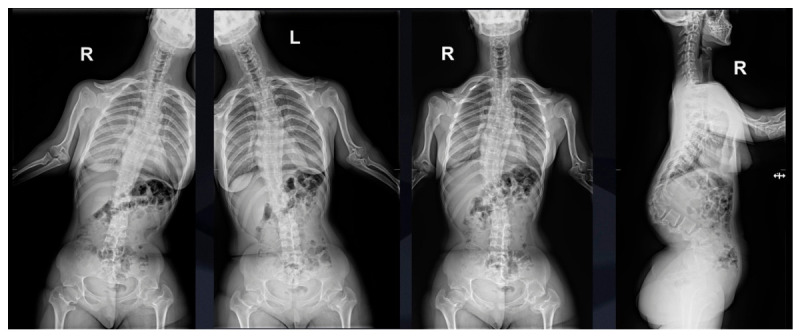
X-ray AP and lateral and bending films of a 16-year-old girl with achondroplasia and congenital severe kyphosis before surgical treatment.
